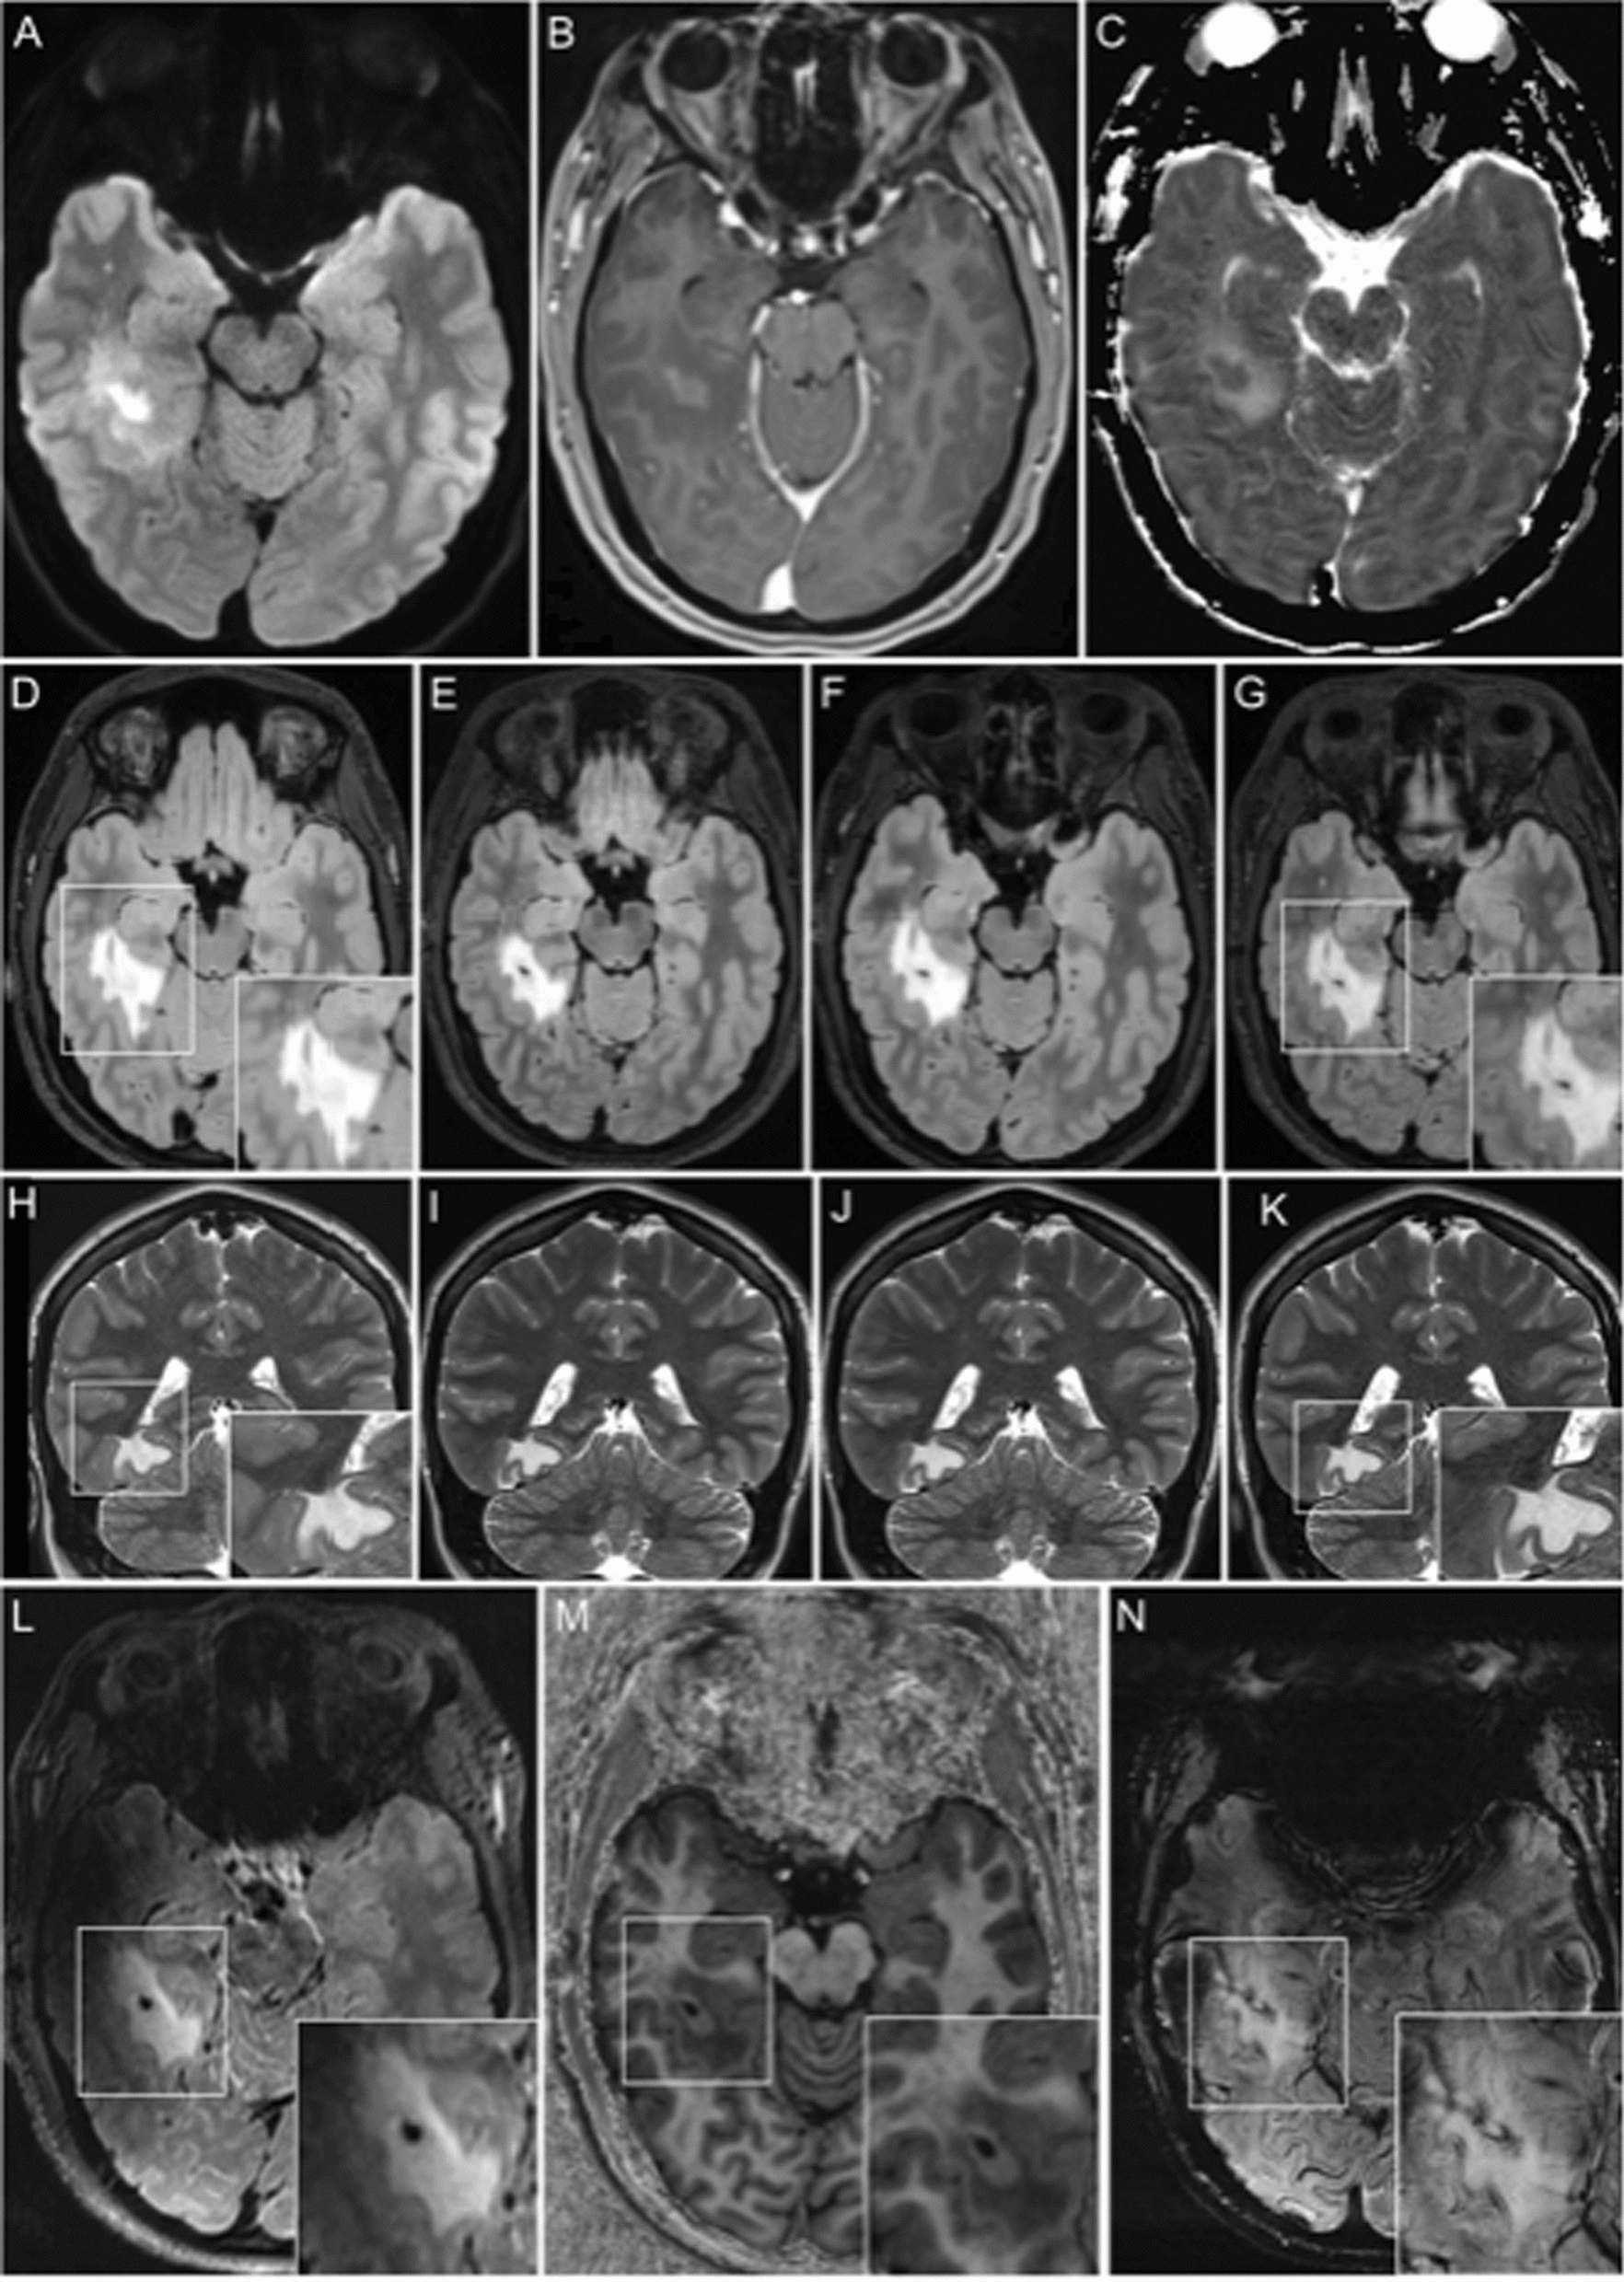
MRI at 3 (A–K) and 7 Tesla (L–N). A–C Initial MRI study. A The FLAIR image shows a hyperintense lesion in the right temporal lobe with a strongly hyperintense center and a perilesional zone of intermediate hyperintensity. B Post-contrast T1 image revealed slight contrast enhancement in the lesion center and T1-hypointensity in the perilesional zone, most likely corresponding to perilesional edema. C Diffusion map with facilitated diffusion in the perilesional area and largely unaltered diffusion metrics in the lesion center. D–G Serial axial FLAIR images and H–K serial coronal T2-weighted images after biopsy show a largely stable lesion which remains strictly confined to the white matter. Two biopsy sites are visible in the lesion. L 7 Tesla FLAIR image reveals a lesion situated in the white matter and partly sparing the subcortical U fibers. M T1-weighted MP2RAGE image identifies a homogeneously hypointense lesion area. The biopsy site is surrounded by an area of increased signal, which might be related to the biopsy procedure. N SWI shows the biopsy site within a lesion of homogenous high signal intensity. The biopsy site itself is characterized by patchy hypointense signal alterations, likely reflecting extravasation of blood products due to the biopsy
